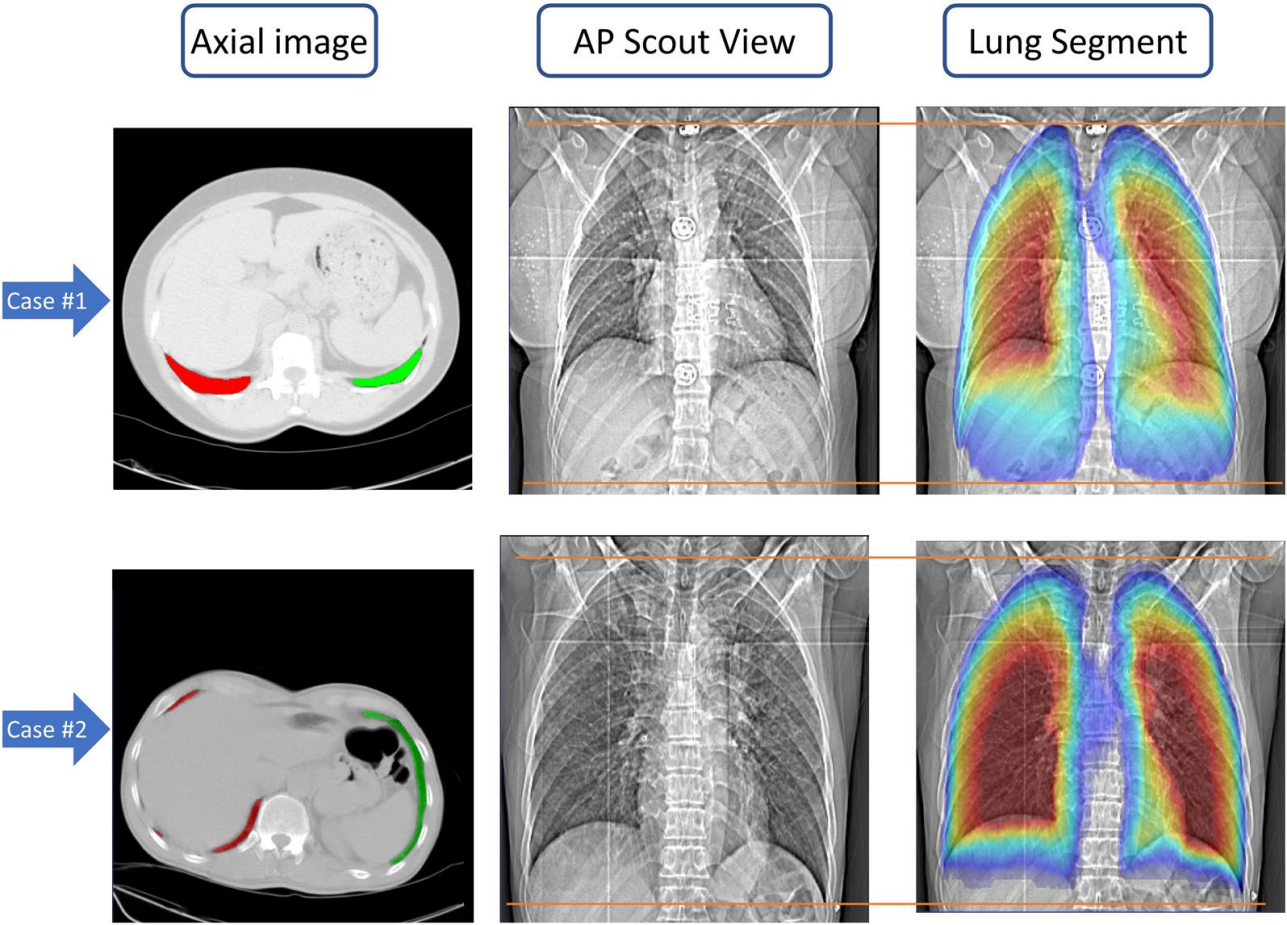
Display of the axial CT image and the segmented lungs (left), AP scout view (middle) and the segmented lung overlaid on the AP scout view (right) for two clinical studies. The red line indicates the desired scan limit in the inferior direction. The colored segments show the thickness of the lung segment in any region, where the red and blue colors stand for the thicker and narrower lung segments, respectively
